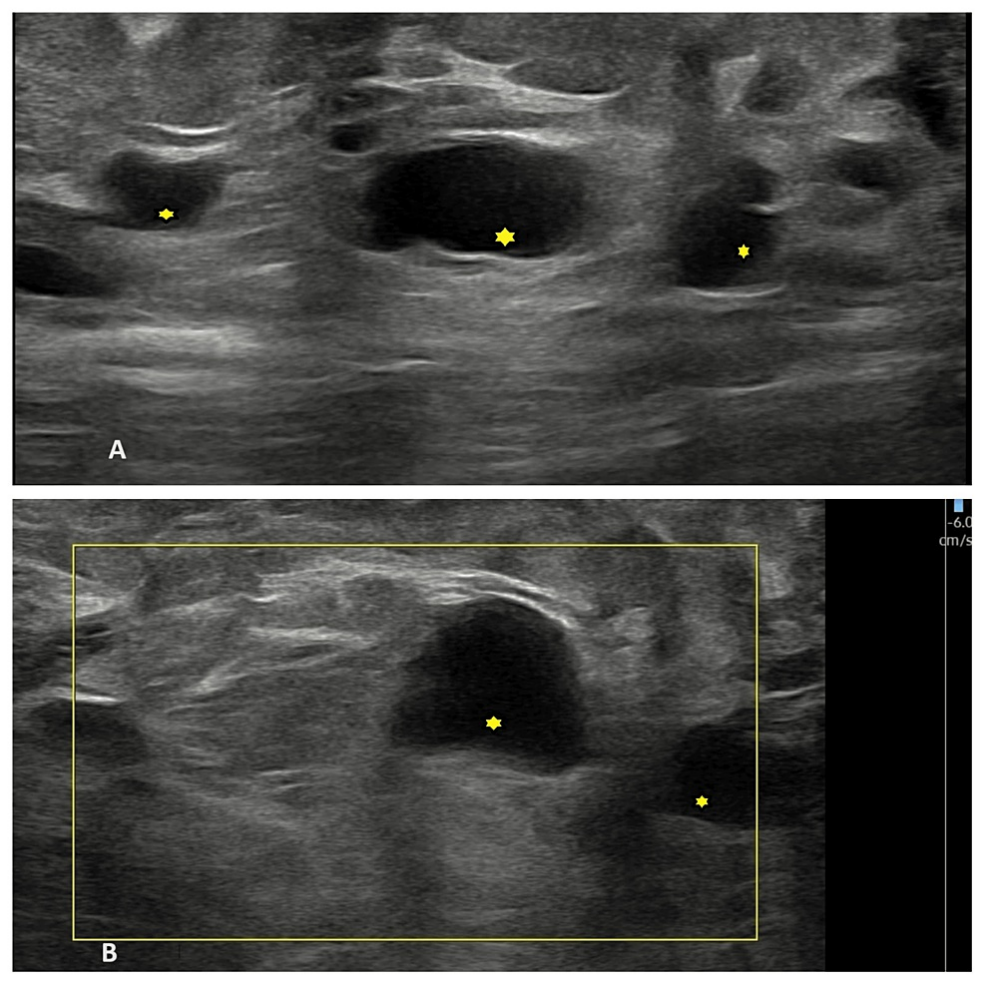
(A): B-Mode ultrasound of the right calf region showing an anechoic multilocular cystic lesion in the subcutaneous plane (indicated by yellow stars). (B): On color Doppler imaging, no vascularity is noted within these cystic spaces (indicated by yellow stars)
